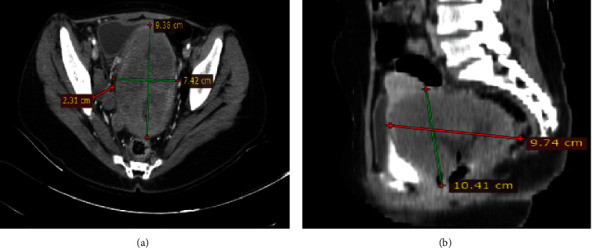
(a) Coronal and (b) sagittal postcontrast CT scans after chemotherapy and pelvic radiation showed a huge abdominopelvic mass extending from the cervicovaginal area and extending to the pelvic side wall, with multiple pelvic lymph nodes, the largest measuring 2 *cm* × 2.5 *cm*, suggesting a recurrent tumor.
